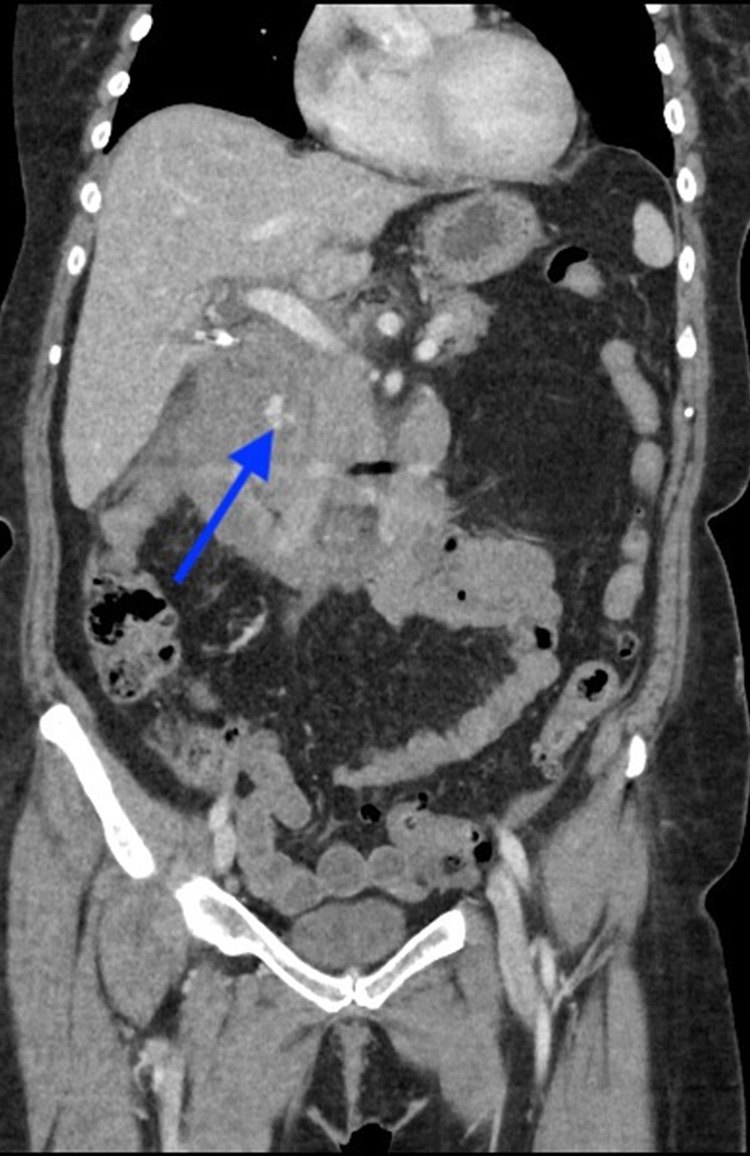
Coronal CT demonstrates a large hematoma in the periduodenal region with a focus of active extravasation (blue arrow).
